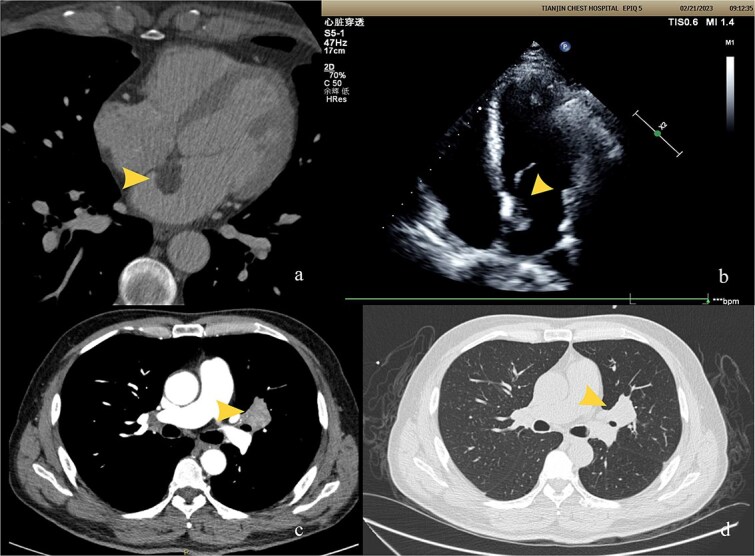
Preoperative imaging. (a) Contrast-enhanced CT showing a left atrial filling defect (arrow). (b) Transthoracic echocardiography demonstrating a pedunculated left atrial mass (arrow). (c, d) Contrast-enhanced CT revealing a left lower lobe hilar mass (arrows), suspicious for primary lung cancer.
